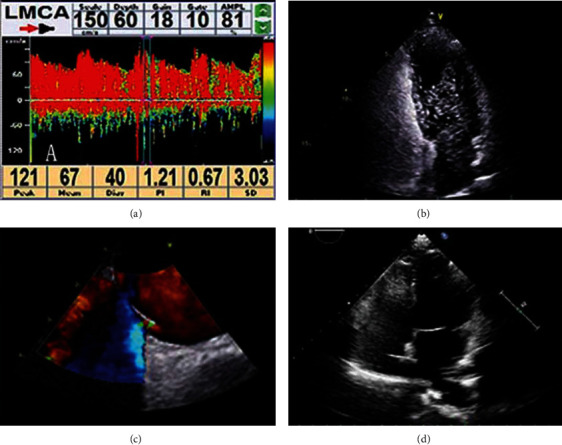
(a) Contrast transcranial Doppler (cTCD) detected large right-to-left shunt in a patient with CS; (b) contrast transthoracic echocardiography (cTTE) showed a large number of microbubbles in the left atrium within three cardiac cycles after the contrast entered the right atrium; (c) transesophageal echocardiography (TEE) detected right-to-left shunt and the width of gap (the yellow arrow) between the atrial septum and PFO valve; (d) contrast transthoracic echocardiography (cTTE) showed no microbubbles in the left atrium after PFO closure.
